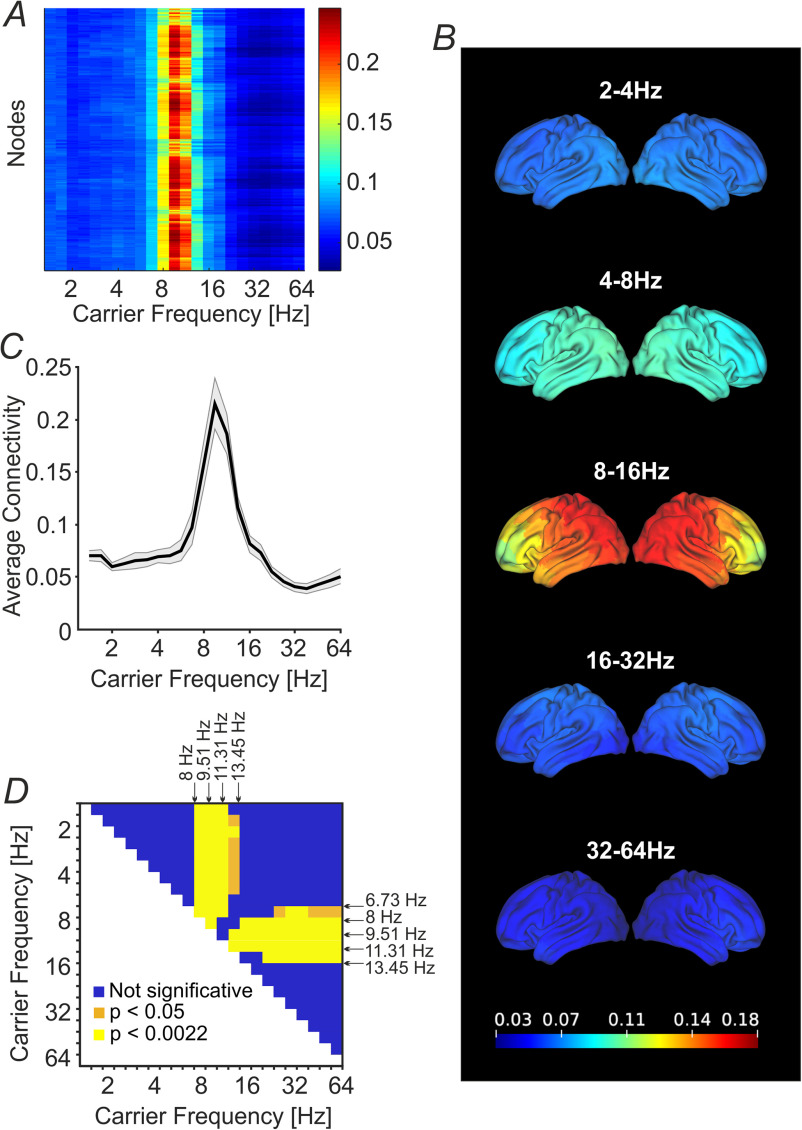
Node strength and average connectivity. (A) Node strength across participants in each wavelet carrier frequency (}{}${2^{({\text{0.5:0.25:6}})}}$Hz), the X-axis is in }{}${\rm{log}_2}$ scale. (B) Node strength frequency specificity overlaid onto the T1-weighted template. For visualization purpose, we further averaged the strength values of the quadruplets between the integer carrier frequencies, obtaining the bands: 2-4 Hz, 4-8 Hz, 8-16 Hz, 16-32 Hz, 32-64 Hz. The colormap is kept fixed to the minimum and maximum values across the bands. See Fig. S1 in the Suppl. Materials where the colormap is customized between minimum and maximum values in each frequency band of interest. (C) The average connectivity is frequency-dependent. Black line and gray shaded-areas indicate mean ± SE across nodes and participants. The X-axis is in }{}${\rm{log}_2}$ scale. (D) Results of the Post-hoc Tukey's HSD test on carrier frequencies. For each couple of frequencies, the lighter elements show a statistically significant difference (yellow: Bonferroni-corrected for multiple comparisons (*p* < 0.0022), orange: uncorrected (*p* < 0.05)). Dark blue elements indicate those frequencies that did not significantly differ among each other. X-axis and Y-axis are in }{}${\rm{log}_2}$ scale.
